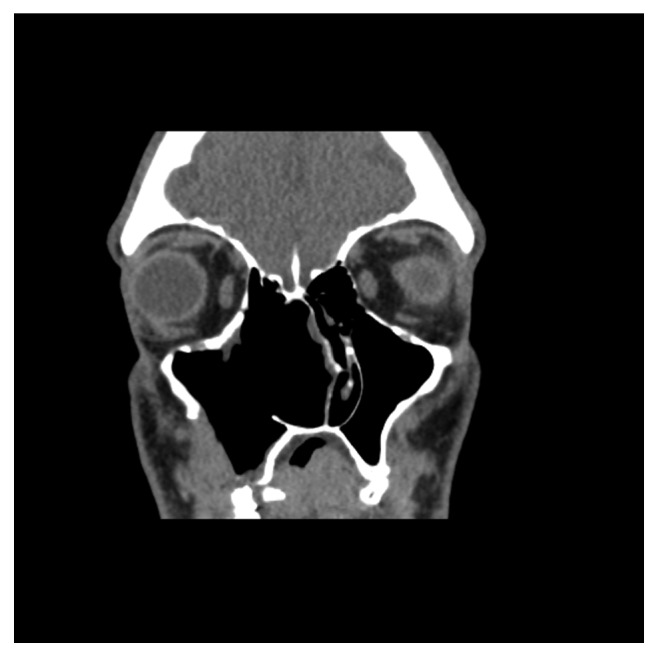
Postoperative CT, coronal plane.
